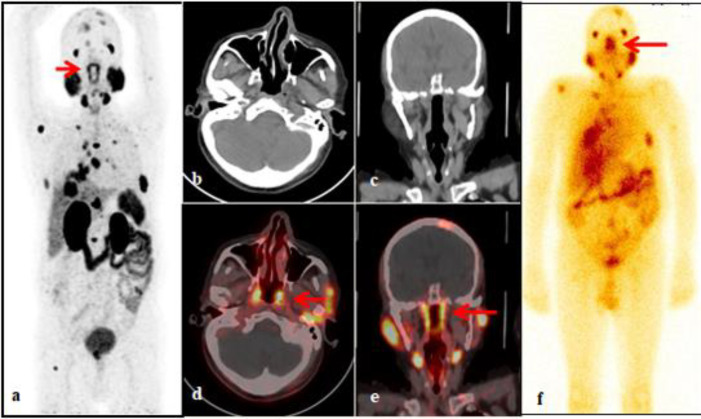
(A-F): ^68^Ga-PSMA PET/CT in a proven carcinoma prostate patient-maximum intensity projection image showing tracer avid lesions in the prostate, multiple skeletal lesions (skull, sternum, scapula, rib, multiple cervicodorsolumbar vertebrae, left iliac bone and right femur), mediastinal lymph nodes, fibro-consolidatory lesion in the lower lobe of the right lung and additional tracer uptake in nasal region (arrow) (a). Axial & coronal CT and corresponding fused PET/CT images of head showing physiological tracer uptake in the major and minor salivary glands, lacrimal glands with additional intense tracer uptake in the region of posterior pharynx likely in the tubarial salivary glands (arrows) (b-e). Anterior post-therapy whole-body image (post ^177^Lu PSMA-617 administration) showing tracer uptake in the tubarial salivary glands (arrow) and at other sites similar to the tracer distribution on ^68^Ga-PSMA PET/CT (f)
